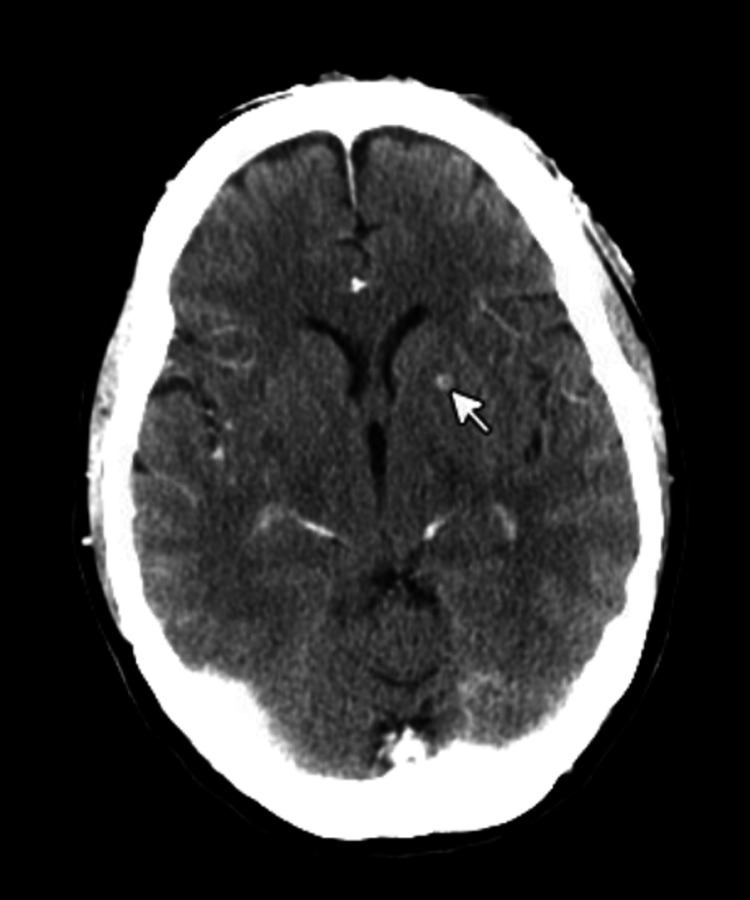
CT of the head. Area of abnormal parenchymal enhancement with central low attenuation within the left lentiform nuclei (white arrow).
